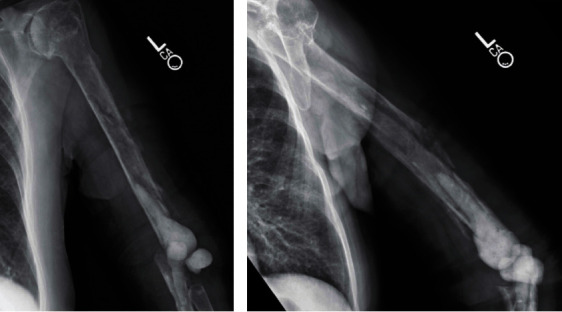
(a, b) Five-month postoperative images following first-stage revision surgery. (a) AP humeral X-rays showing delayed healing of the humeral shaft fracture and antibiotic spacer of the humerus, ulna, and elbow joint. (b) Lateral humerus X-ray showing incomplete healing of the humeral shaft fracture.
